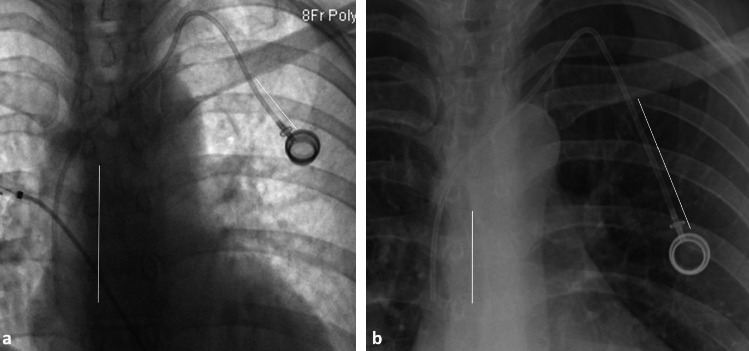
Left-sided port measurements. (a) Magnified fluoroscopic image of initial left-sided port insertion demonstrating typical measurement locations (solid white lines). (b) Magnified follow-up chest radiograph demonstrating typical measurement locations (solid white lines). This example is a 45-year-old female with a left-sided port in situ and 3-day interval between imaging. Δ catheter tip-to-carina: −20.3 mm (cephalad, retracted); Δ port: +31.6 mm (caudal, protracted); zone on fluoroscopy: upper right atrium (Zone A); zone on follow-up: distal superior vena cava (Zone A).
